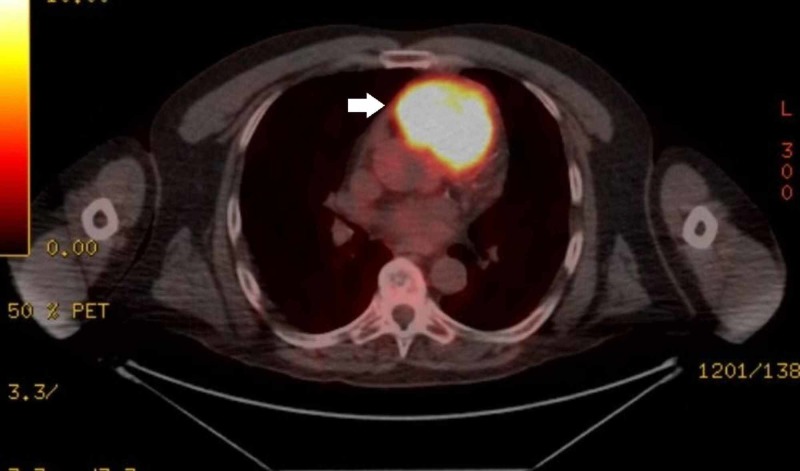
F-fluorodeoxyglucose-positron emission tomography (FDG-PET) revealing an intensely hypermetabolic soft tissue mass within the right ventricle (arrow) measuring 6.9 x 6.2 cm.
